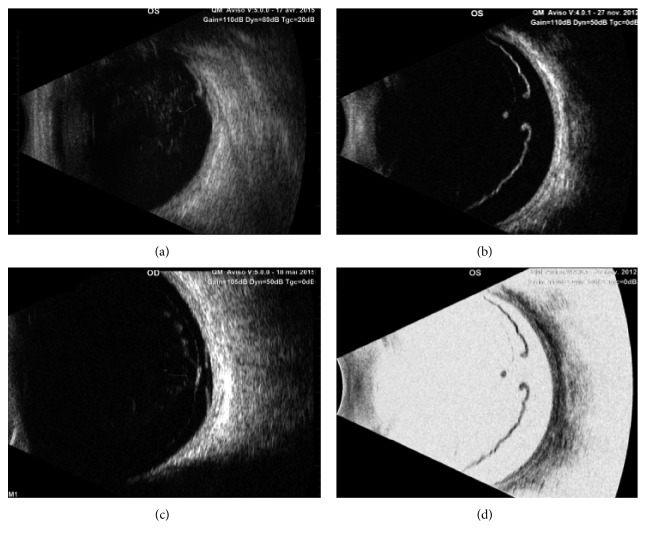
B-scan ultrasonography: (a) Eleven o'clock meridian acquired with a 10 MHz probe (Aviso, Quantel Médical) showing a typical retinal tear on B-scan ultrasonography. (b) Superior longitudinal scan acquired with a 20 MHz probe showing a typical retinal tear on B-scan ultrasonography. (c) Superior longitudinal scan acquired with a 20 MHz probe showing a retinal tear associated with retinal detachment. (d) Same pictures than in C with reversed grey scale for a better visualization of the retina and underlying tissue.
